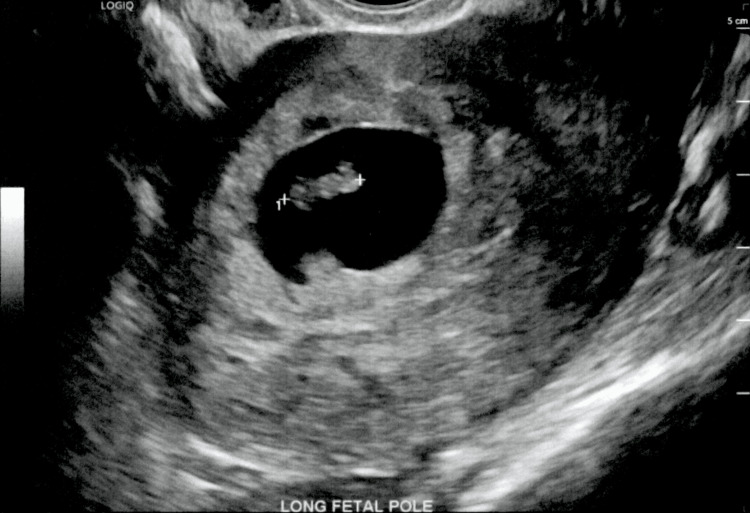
TVUS of the long fetal pole showing an intrauterine gestation with the crown-rump length measuring 9.8 mm TVUS: transvaginal ultrasound
